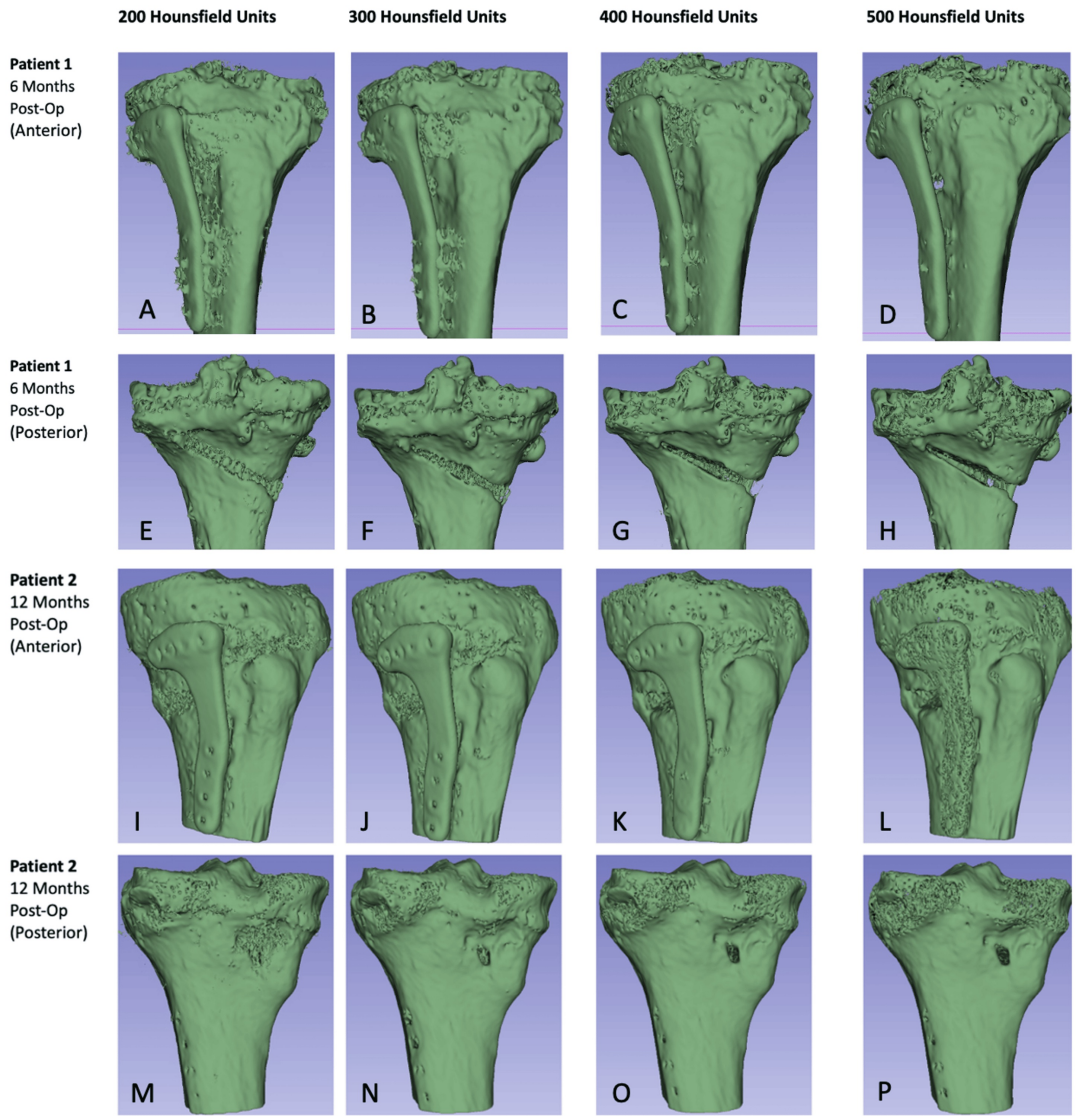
Postoperative CT 3D reconstruction scans at 6 and 12 months. A: six months postoperative anterior view of CT 3D reconstruction at 200 HU; B: six months postoperative anterior view of CT 3D reconstruction at 300 HU; C: six months postoperative anterior view of CT 3D reconstruction at 400 HU; D: six months postoperative anterior view of CT 3D reconstruction at 500 HU; E: six months postoperative posterior view of CT 3D reconstruction at 200 HU; F: six months postoperative posterior view of CT 3D reconstruction at 300 HU; G: six months postoperative posterior view of CT 3D reconstruction at 400 HU; H: six months postoperative posterior view of CT 3D reconstruction at 500 HU; I: 12 months postoperative anterior view of CT 3D reconstruction at 200 HU; J: 12 months postoperative anterior view of CT 3D reconstruction at 300 HU; K: 12 months postoperative anterior view of CT 3D reconstruction at 400 HU; L: 12 months postoperative anterior view of CT 3D reconstruction at 500 HU; M: 12 months postoperative posterior view of CT 3D reconstruction at 200 HU; N: 12 months postoperative posterior view of CT 3D reconstruction at 300 HU; O: 12 months postoperative posterior view of CT 3D reconstruction at 400 HU; P: 12 months postoperative posterior view of CT 3D reconstruction at 500 HU.
